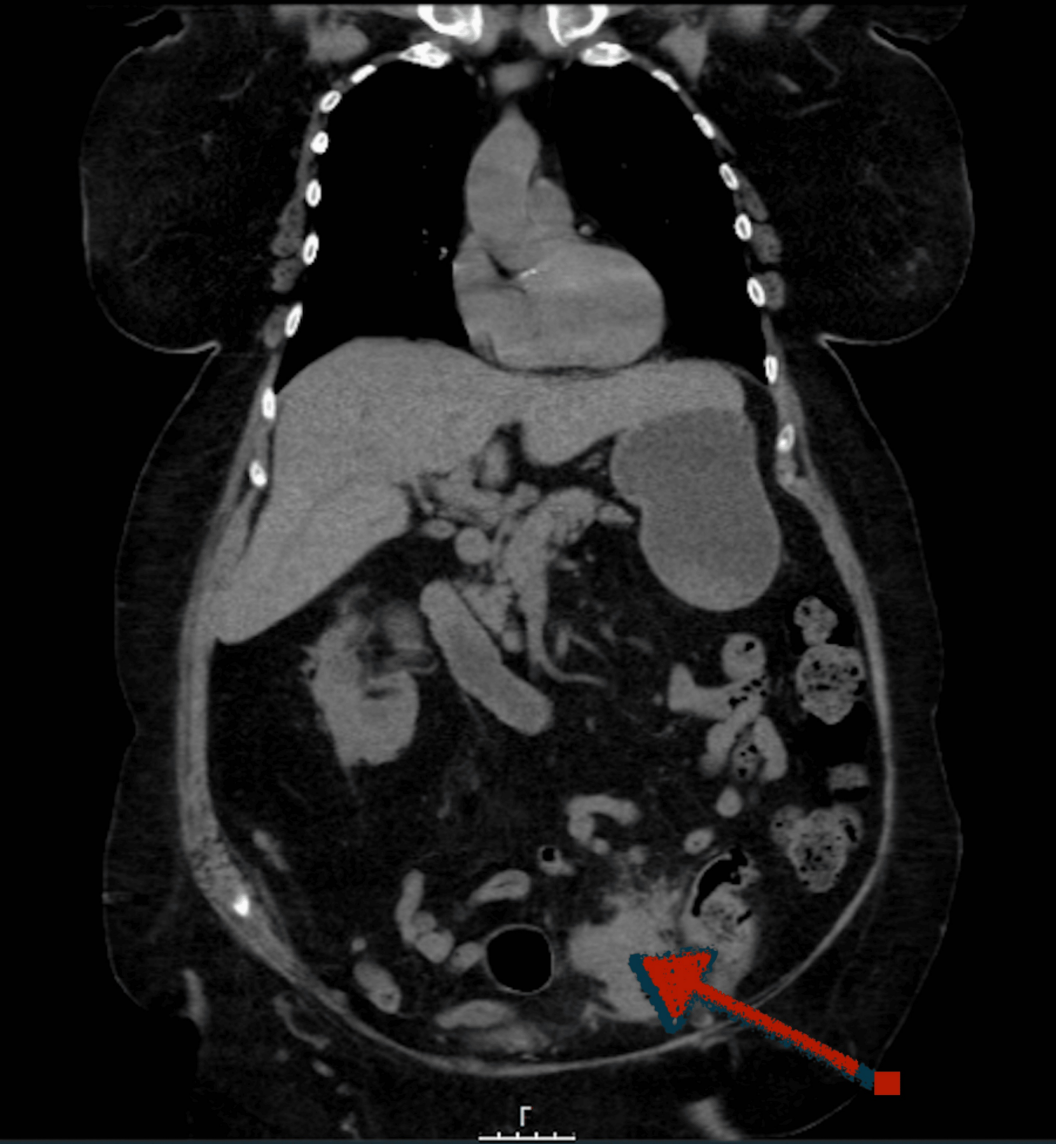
Coronal section of the CT chest-abdomen-pelvis, showing sigmoidal mass nearly obstructing the bowel (red arrow) CT: computed tomography
